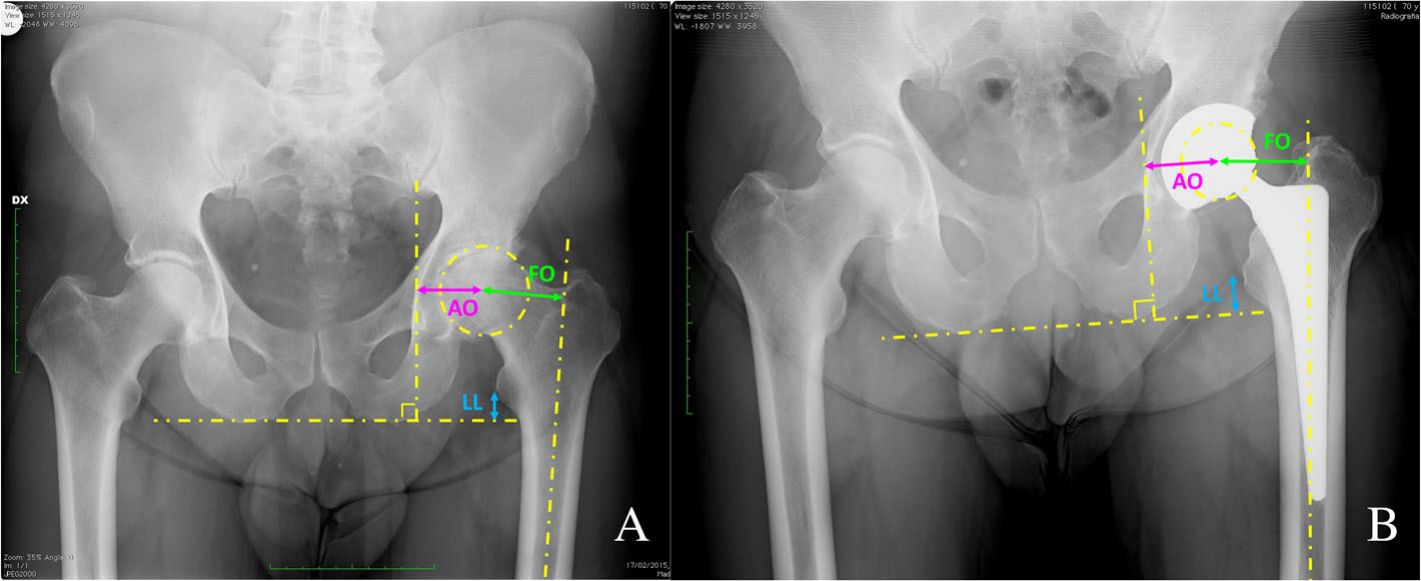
Measurements of the hip biomechanical radiographic parameters by using Horos Viewer software for MAC at preoperative (a) and follow-up time (b). The global hip offset (GO) was defined by the sum of the femoral offset (FO) and the acetabular offset (AO). Limb length (LL) was defined as the distance between the medial apex of the ipsilateral lesser trochanter and the line passing through the lower margins of the ischial tuberosity
